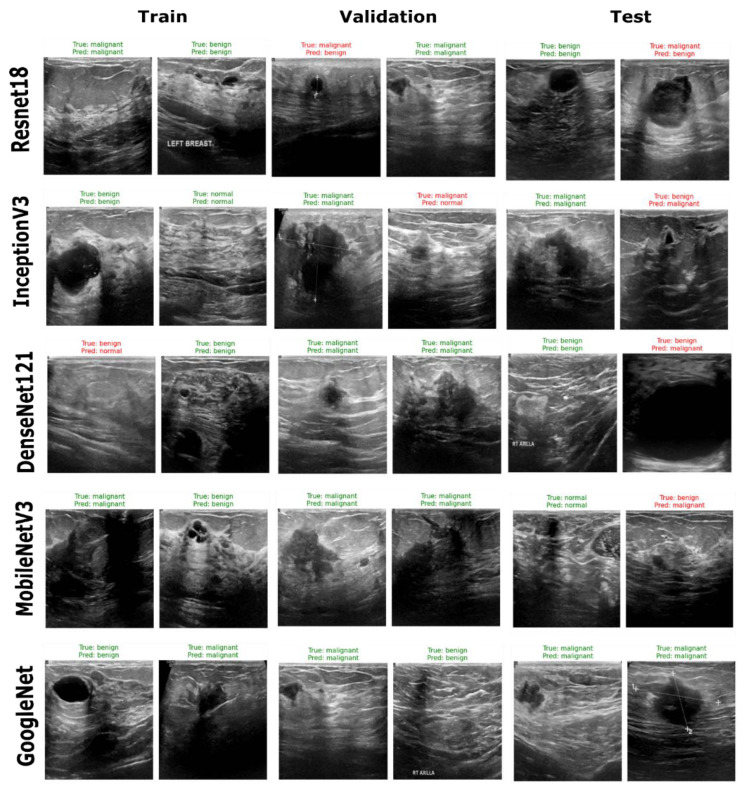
Samples of classification performance of five encoder architectures (ResNet18, InceptionV3, DenseNet121, MobileNetV3 and GoogleNet) across training, validation and test datasets. Green labels indicate correct predictions, while red labels denote misclassifications. The figure demonstrates each model’s ability to discriminate between normal, benign and malignant breast lesions in ultrasound images.
